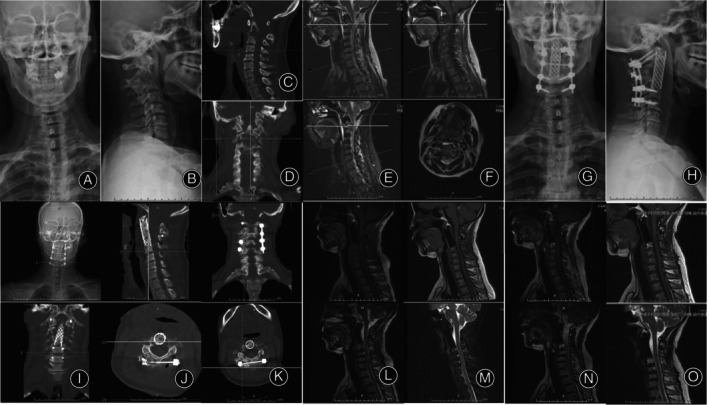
Male, 51 years old, with plasmacytoma. (A,B) Preoperative x‐rays showing lesions in the C2 vertebra. (C,D) Preoperative CT scans revealing bone destruction in the C2 vertebra. (E,F) Preoperative lumbar spine MRI demonstrating C2 vertebral bone destruction. (G,H) The x‐ray taken 1 week postoperatively showed that the cervical pedicle screws and the titanium cage were stable. (I,J) The CT scan 1 week postoperatively showed adequate spinal canal decompression, with the titanium cage and pedicle screws remaining stable. (K) The CT scan taken 6 months postoperatively revealed adequate decompression of the spinal canal, with the titanium cage and pedicle screws remaining stable. (L) The MRI taken 6 months postoperatively showed no significant tumor recurrence in the surgical area, with the titanium cage and pedicle screws remaining stable. (M) The MRI taken 1 year postoperatively showed no significant tumor recurrence in the surgical area, with the titanium cage and pedicle screws remaining stable. (N) The MRI taken 2 years postoperatively showed no significant tumor recurrence in the surgical area, with the titanium cage and pedicle screws remaining stable. (O) The MRI taken 34 months postoperatively showed no significant tumor recurrence in the surgical area, with the titanium cage and pedicle screws remaining stable. CT, computed tomography; MRI, magnetic resonance imaging.
